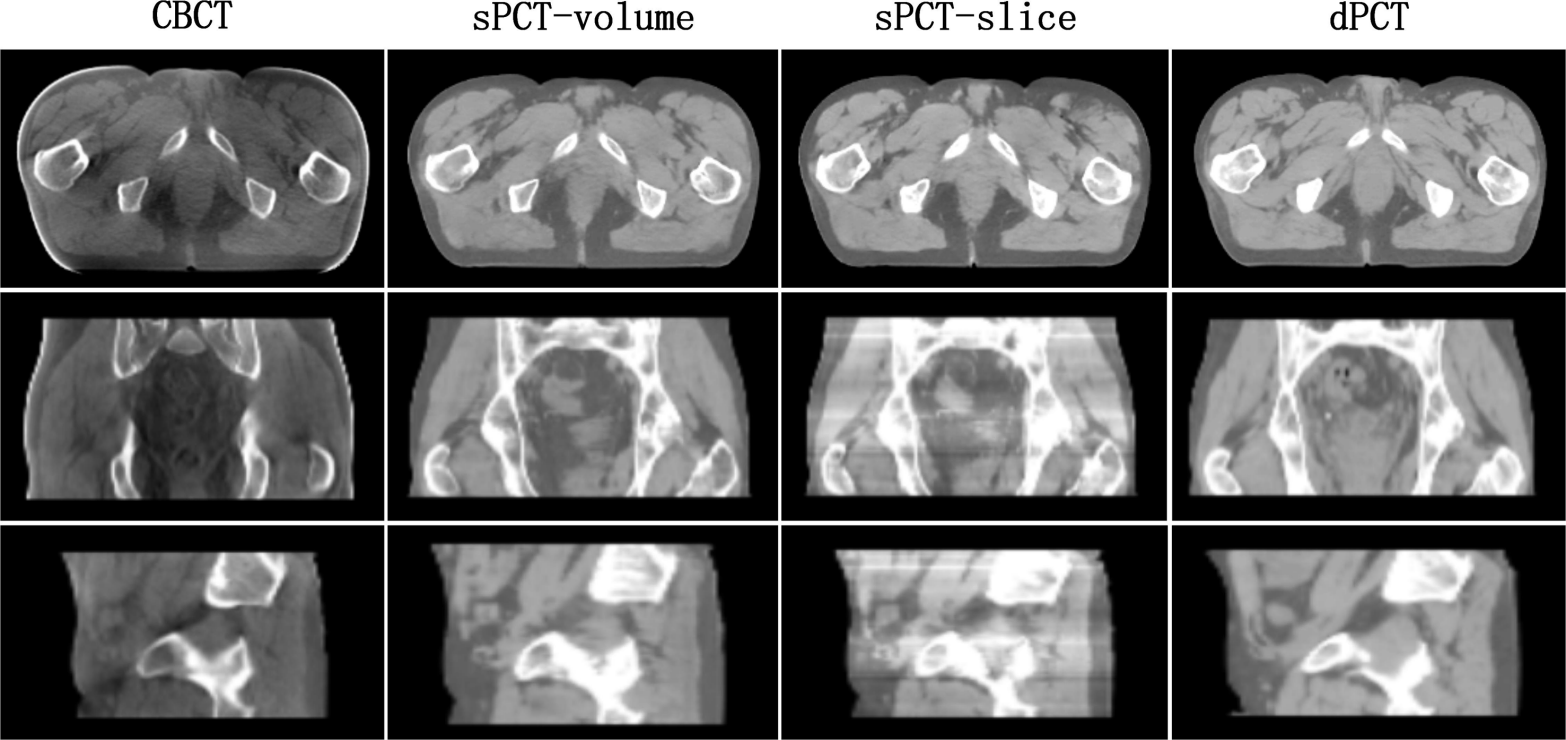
Effect of different normalization method on sPCT. Each column from left to right represents CBCT, sPCT (3D), sPCT (2D), and dPCT respectively. The top, middle, and bottom rows represent axial, coronal, and sagittal images respectively. Strip artifacts were found in the coronal and sagittal planes of 2D sPCT images. The display window is (700, 1300) HU.
